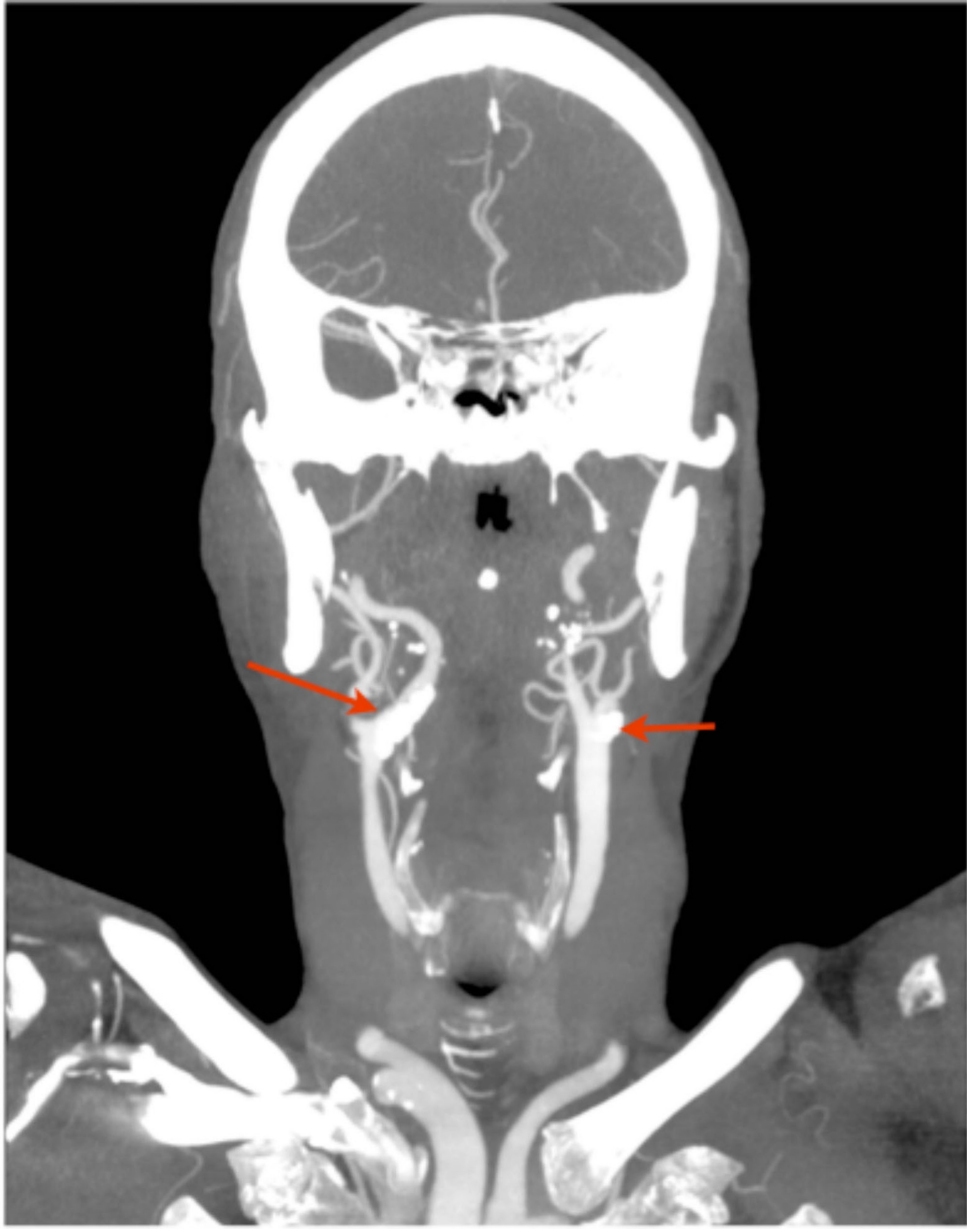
CT angiogram. Scan taken 10 days post-admission. The scan shows calcified plaques involving both carotid bifurcations larger on the right than the left. CT: computed tomography
